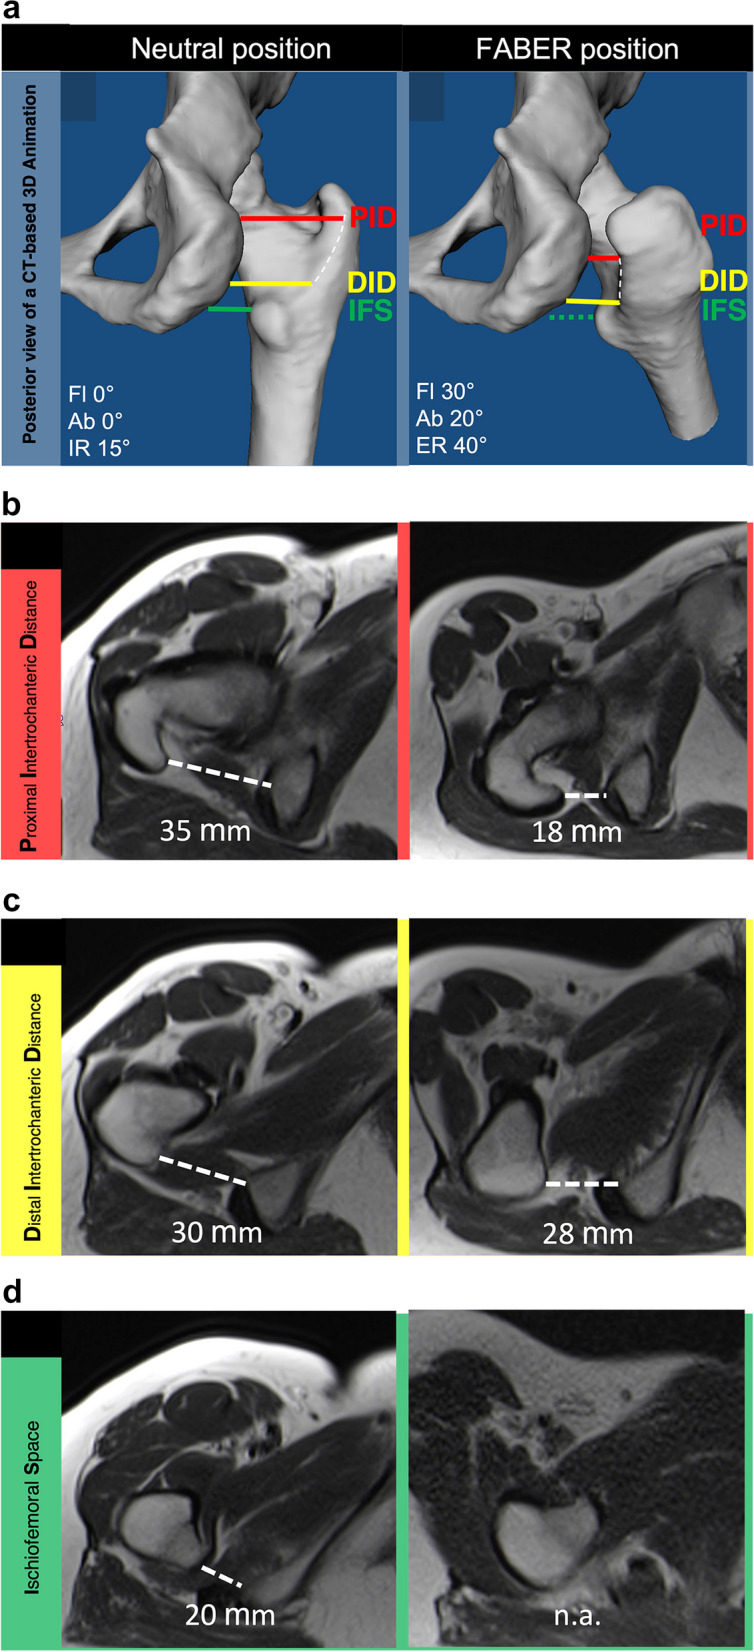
a Posterior view of a CT-based 3D animation of a right hip joint in neutral (left) and FABER (right) positions with the corresponding MRI images measuring the (b) proximal intertrochanteric distance (PID, red line) and (c) distal intertrochanteric distance (DID, yellow line), as well as the (d) ischiofemoral space (IFS, green line). The white dashed line in the 3D animation marks the intertrochanteric ridge. Fl, flexion. Ab, abduction. IR, internal rotation. ER, external rotation. n.a.,= not applicable
